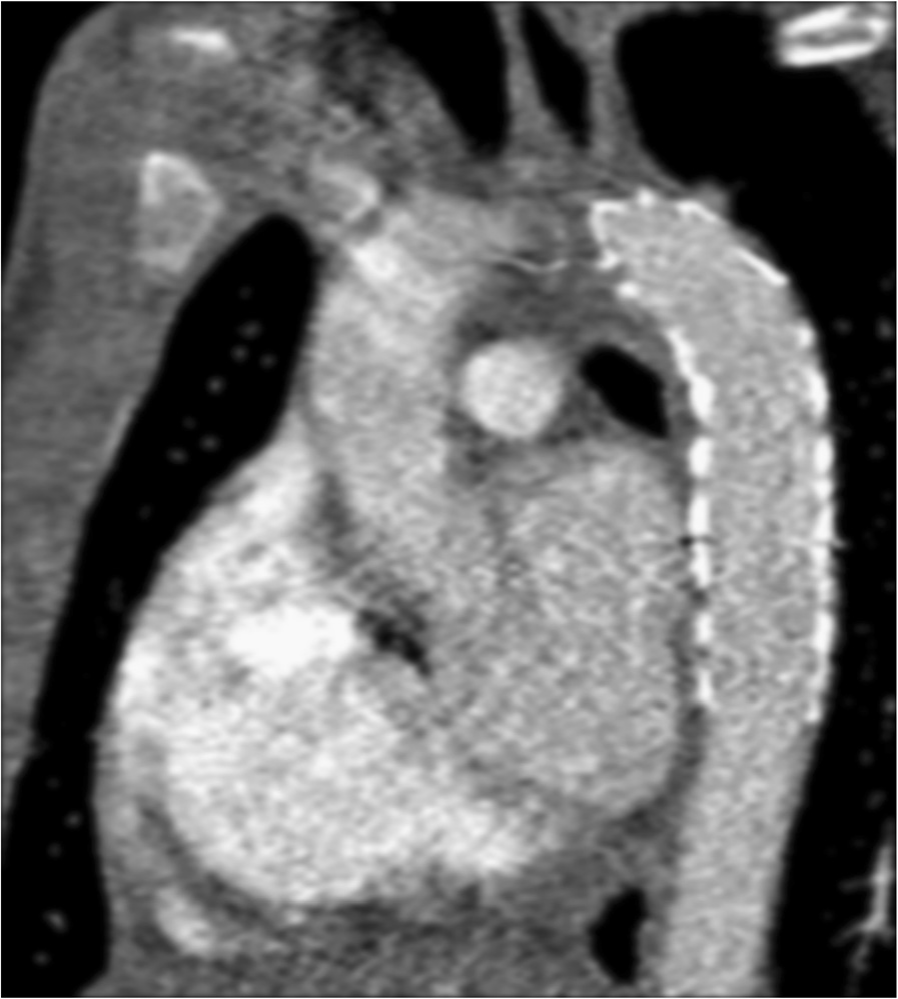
CT image following endograft placement demonstrating seal of aortic injury.
